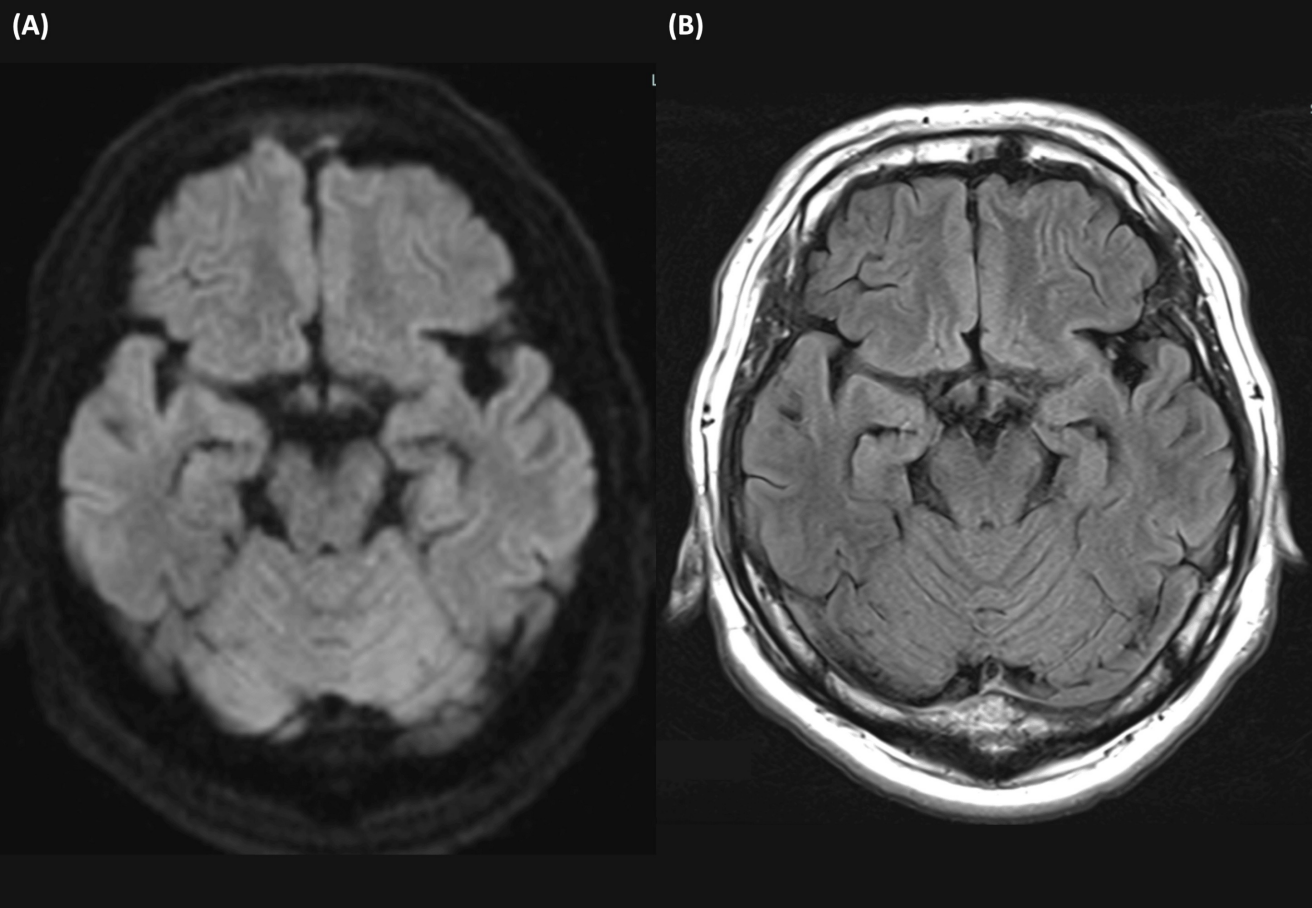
Brain magnetic resonance imaging (MRI) findings. Axial diffusion-weighted imaging (DWI) (A) and fluid-attenuated inversion recovery (FLAIR) imaging (B) obtained on admission. The scans demonstrate normal signal intensity and volume in the medial temporal lobes (hippocampi) and other regions.
